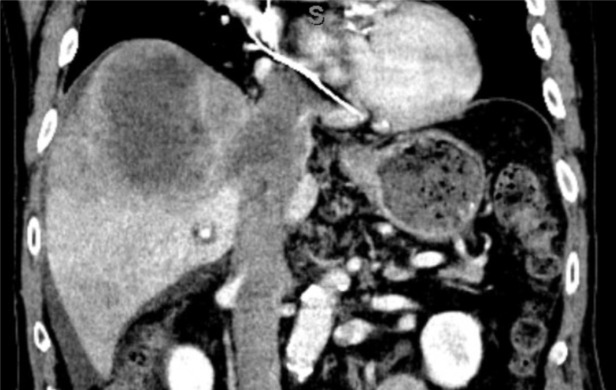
Chest/abdomen computed tomography (CT) with contrast (coronal view) shows a mass in the liver dome with extensive vascular invasion.
